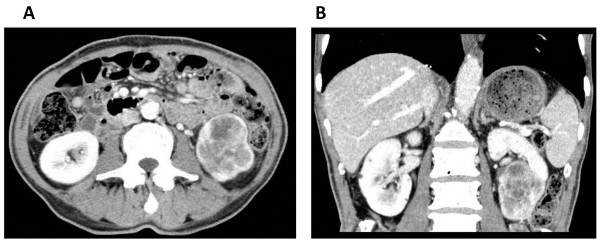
Abdominal enhanced computed tomography (CT) after axitinib treatment. (A, axial section; B, coronal section). Abdominal CT shows a hypervascular tumor (size, 4.5 × 2.5 cm) in the lower pole of the left kidney. The tumor diameter shrank by 56%, compared to that before axitinib treatment.
